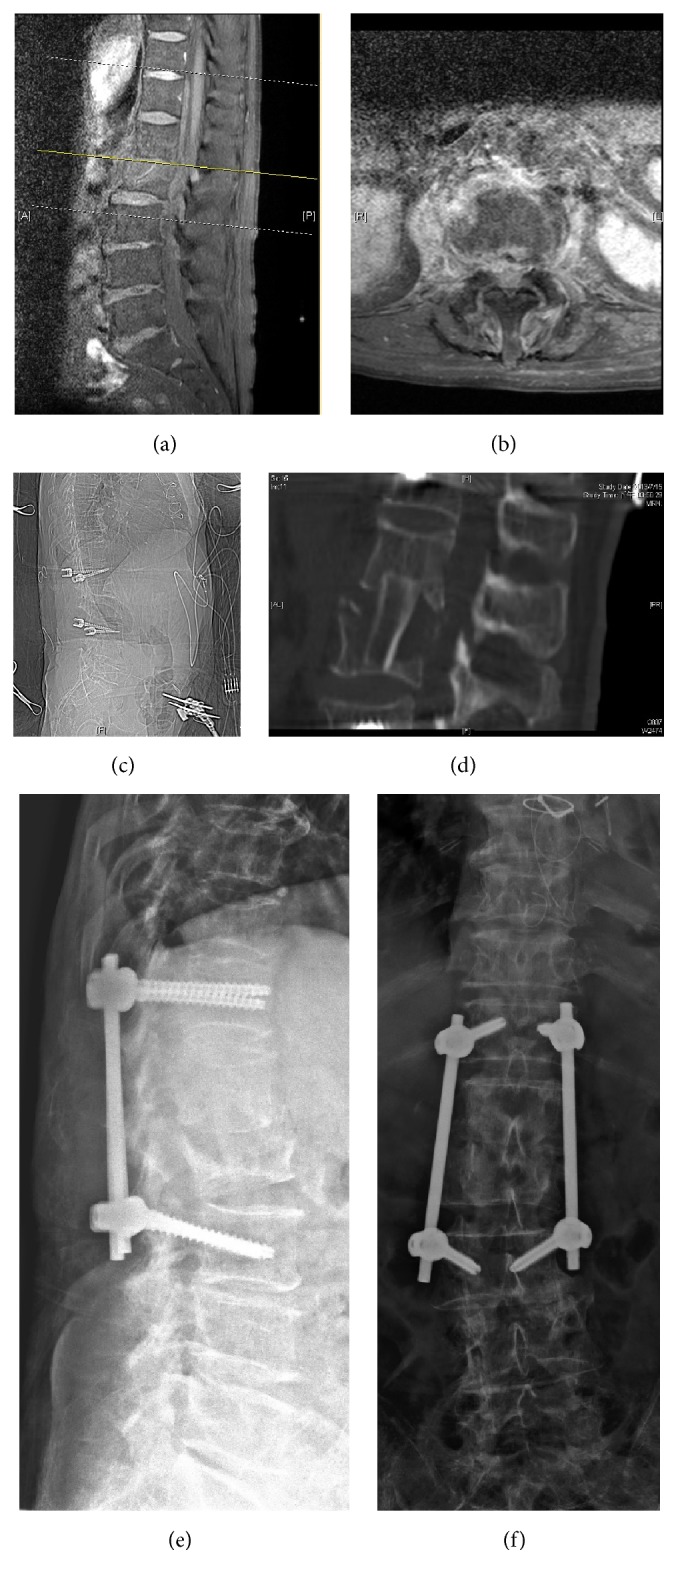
L1-L2 infectious spondylitis accompanied with epidural abscess treated with simultaneous minimally invasive anterior and posterior surgery using the iCT-guided navigation. Preoperative MRI demonstrated L1-L2 vertebral osteomyelitis and epidural abscess with obvious canal compromise (a, b). Intraoperative CT image obtained following simultaneous anterior and posterior spinal surgery (c, d) showing the spinal decompression and reconstruction. Partial resection of the L2 vertebra with reconstruction of L1-L2 with a percutaneous pedicle screw-rod construct and interbody iliac strut bone grafting (e, f).
